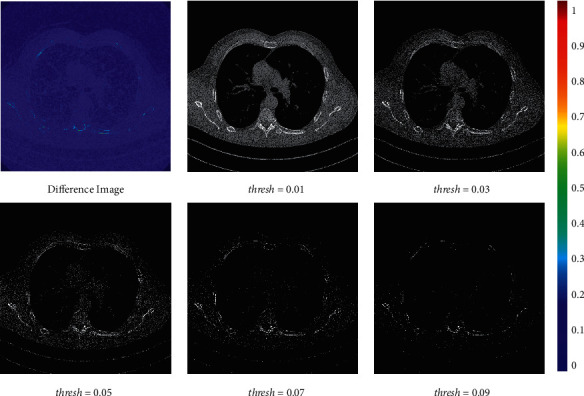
The difference image and filtered normal-dose CT images using different threshold values. The difference image was processed by pseudocolorization technology for better visualization. The display window is [0, 400] Hu for filtered normal-dose CT images.
